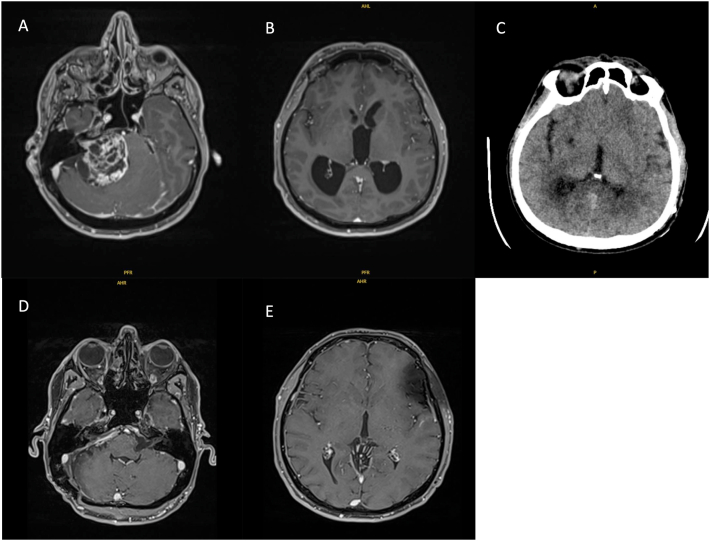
Axial T1-weighted magnetic resonance imaging (MRI) showing (A) a 5,3 cm gadolinium-enhancing tumor within the right cerebellopontine angle (CPA) and (B) hydrocephalus. (C) Axial CT scan after ventriculoperitoneal shunt to treat hydrocephalus. (D) A post operative axial MRI confirmed total removal of tumor and (E) regression of hydrocephalus one year later.
